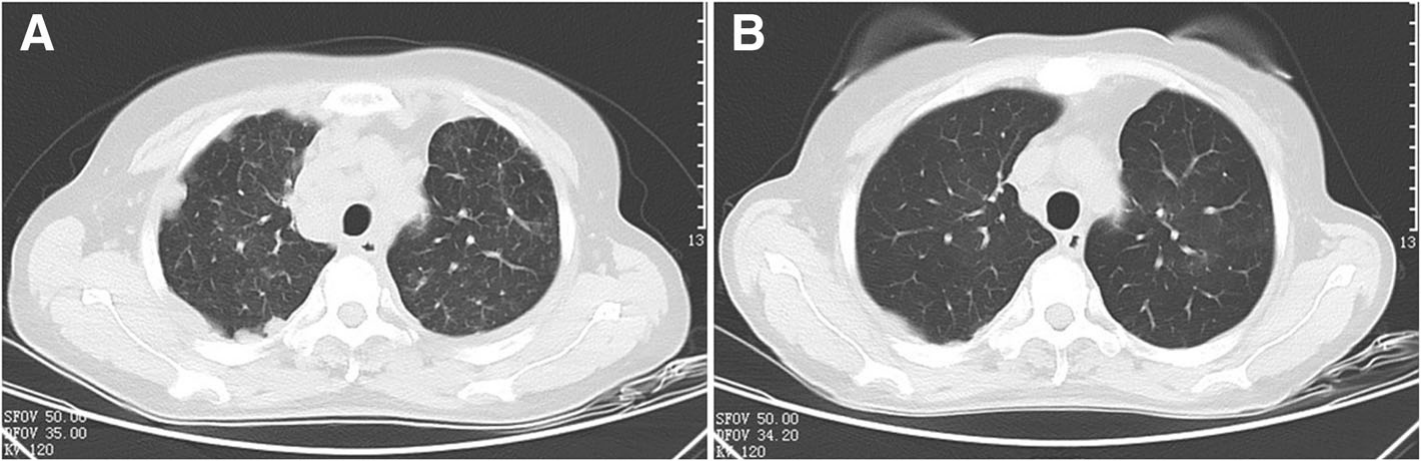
Computed tomography (CT) scans before (a) and after (b) crizotinib therapy
